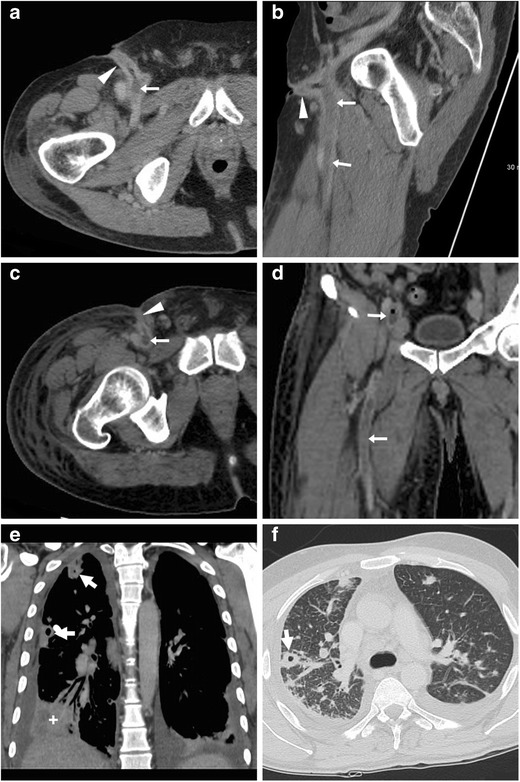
Two different cases of septic thrombophlebitis (STP) after intravenous drug injection. A-B) in a 57-year-old HIV-seropositive male patient, contrast-enhanced CT images showed strongly enhancing “tram-track” fistula (arrowheads) along the puncture track, and similar mural enhancement of the thrombosed ipsilateral femoral vein (arrows). Pus culture from draining orifice tested positive for Methycillin-sensitive *Staphylococcus aureus*. C-F) in a 34-year-old man with high fever, right thigh swelling, bloody and purulent drainage at the groin. The non-opacified right common, external iliac and femoral veins (arrows in C, D) showed prominent wall enhancement and some endoluminal gas bubbles. A “tram-track” fistula connected to the depressed injection site (arrowhead in C). Additionally, body CT showed pleural effusions, lung base consolidation (+), mediastinal adenopathies and subpleural lesions with central cavitations consistent with septic emboli (thick arrows in E, F). Blood and groin pus cultures revealed polymicrobial infection, which required two months of antibiotics and intensive care hospitalisation [Adapted with permission from ref. no. [39]]
